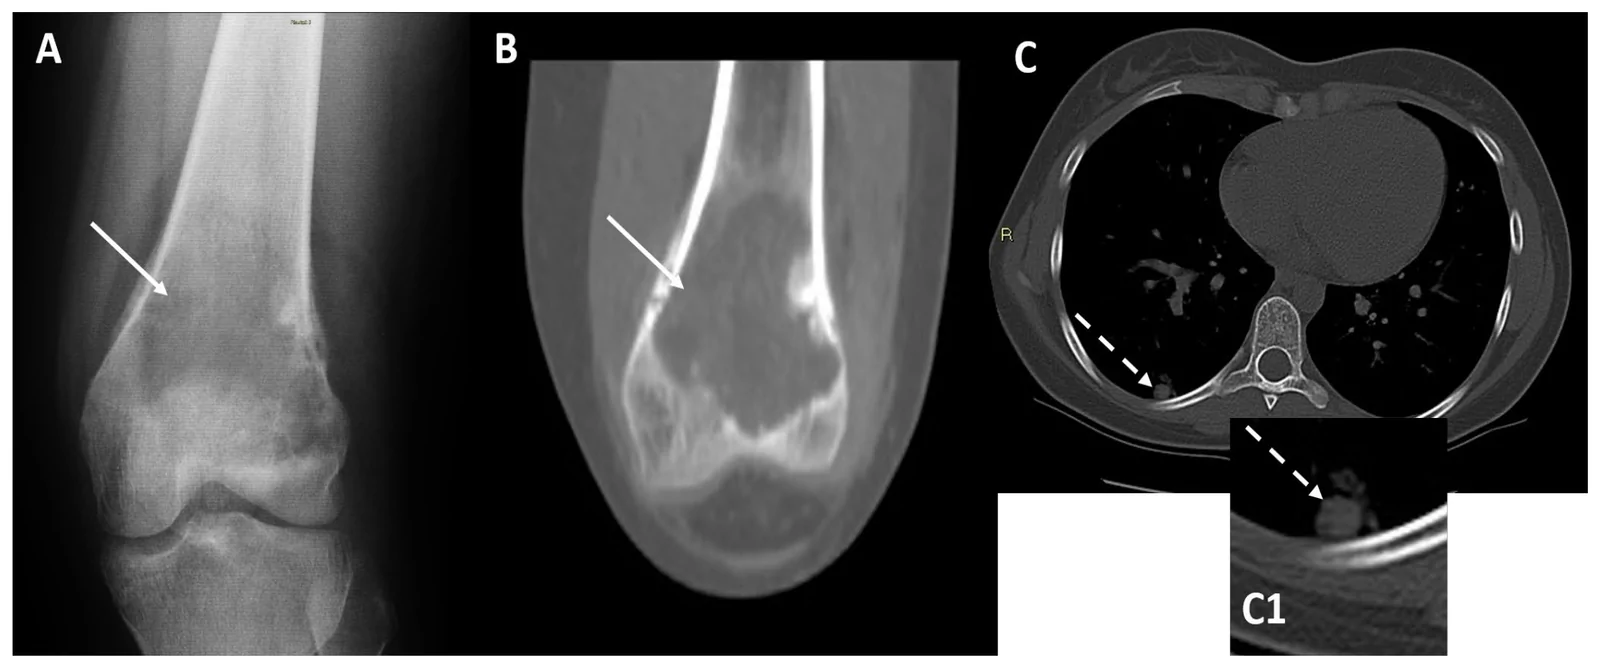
A 16-year-old girl affected by telangiectatic osteosarcoma of the left distal femur. Panel (A) (conventional radiology) and Panel (B) (CT with coronal reconstruction) show a purely lytic radiologic patter-; Panel (C) (Baseline CT with bone window; magnified in (C1)) shows lung metastases without internal calcification. Lesions are indicated by arrows.
